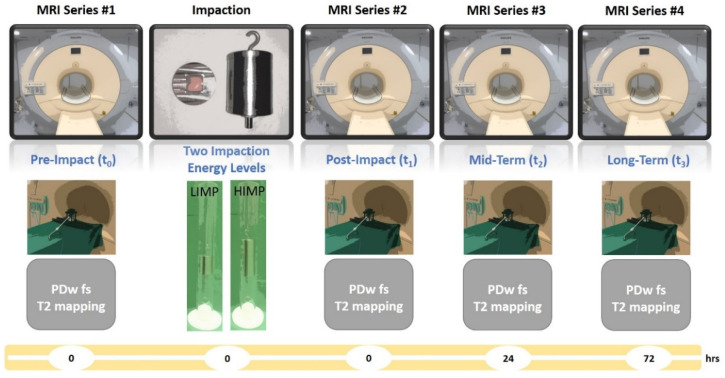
MRI series timeline. Cartilage samples were imaged immediately before (t_0_) and after impaction (t_1_) as well as after another 24 h (t_2_) and 72 h (t_3_). The imaging protocol was completed for each MRI series (grey boxes). Unit of timeline (yellow) is hours.
